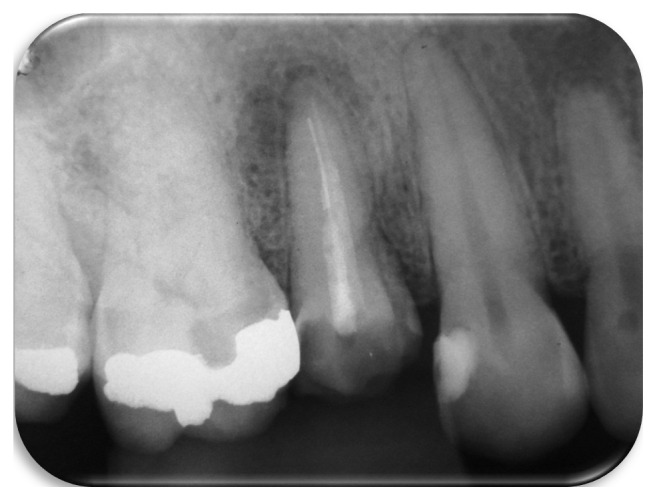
Initial periapical radiograph showing the fragment at the apical canal third.
